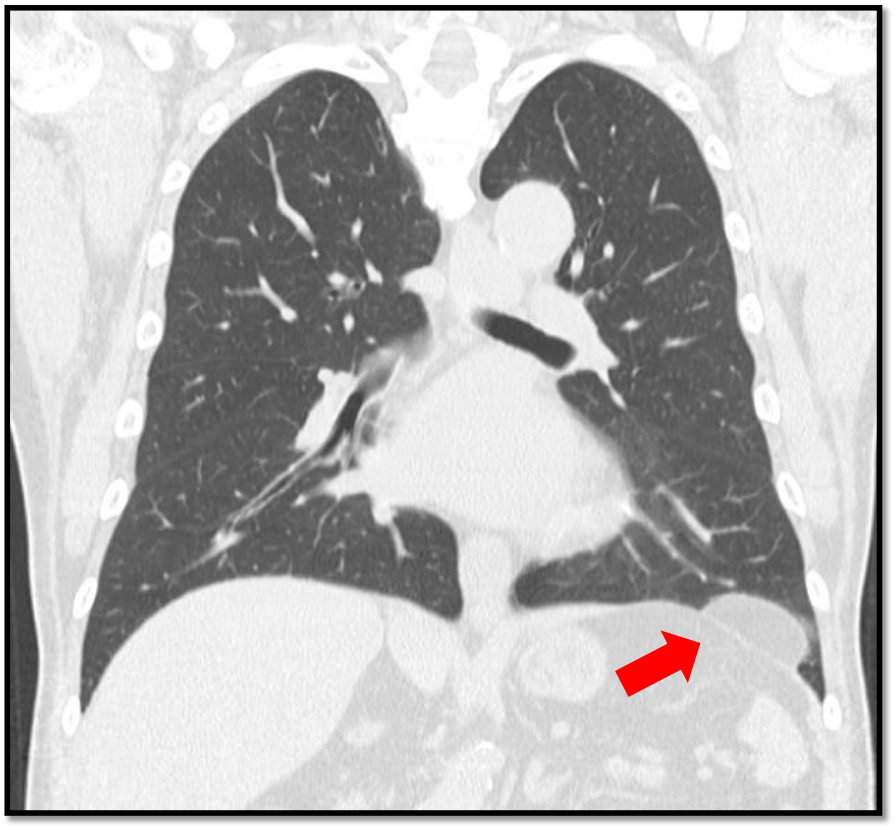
Chest computed tomography (CT) showed a 5-cm solid tumor on the left diaphragm
